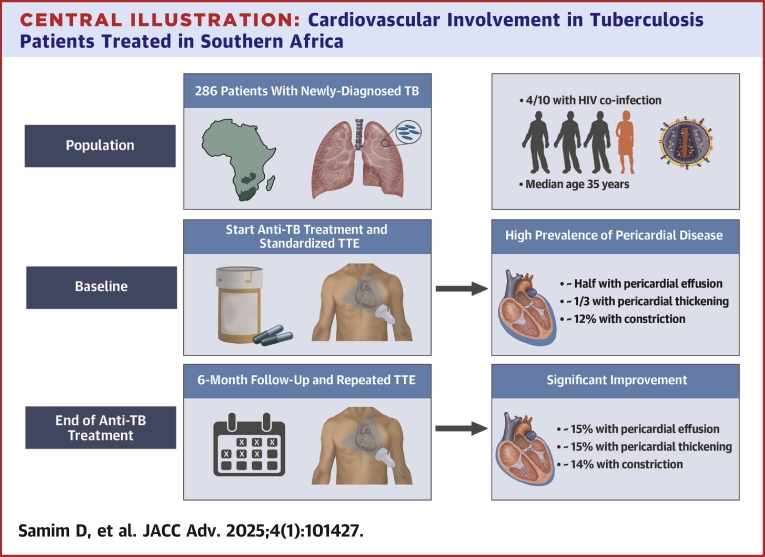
Cardiovascular Involvement in Tuberculosis Patients Treated in Southern Africa TTE = transthoracic echocardiography; TB = tuberculosis.
